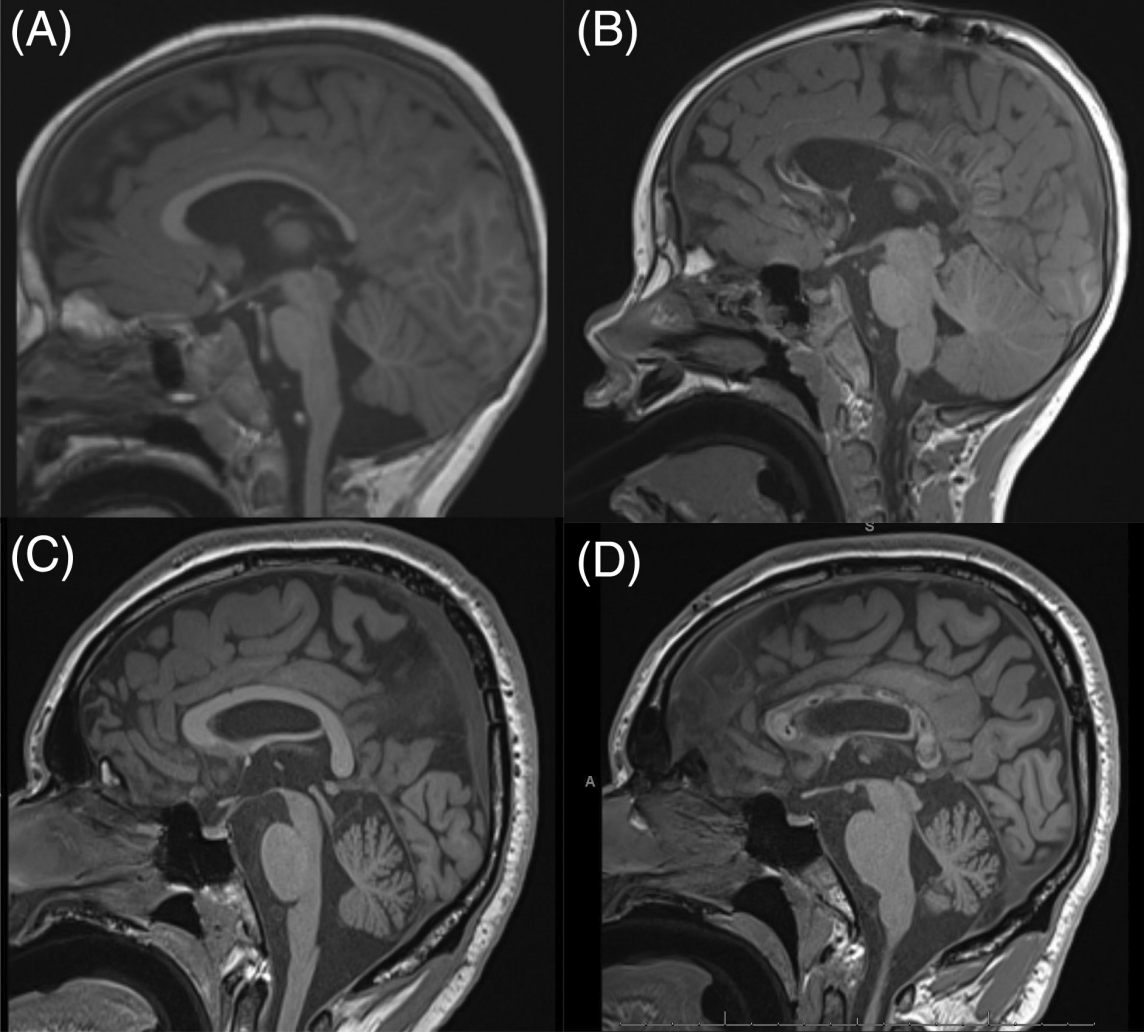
T1‐weighted MRI demonstrated pre‐ and postoperative imaging of patients undergoing open callosotomy (Panels A and B) and corpus callosum ablation (Panels C and D).
